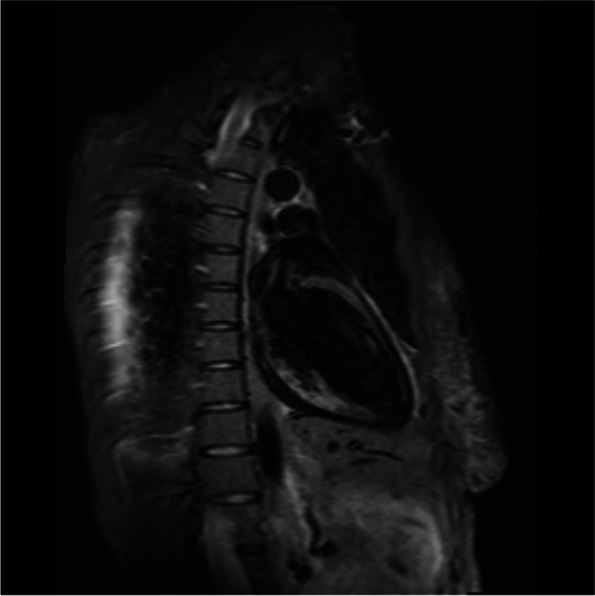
Cardiac MRI, T2-weighted stir sequence, long-axis view, showing an increased signal intensity of the mid inferior and basal inferior segment
